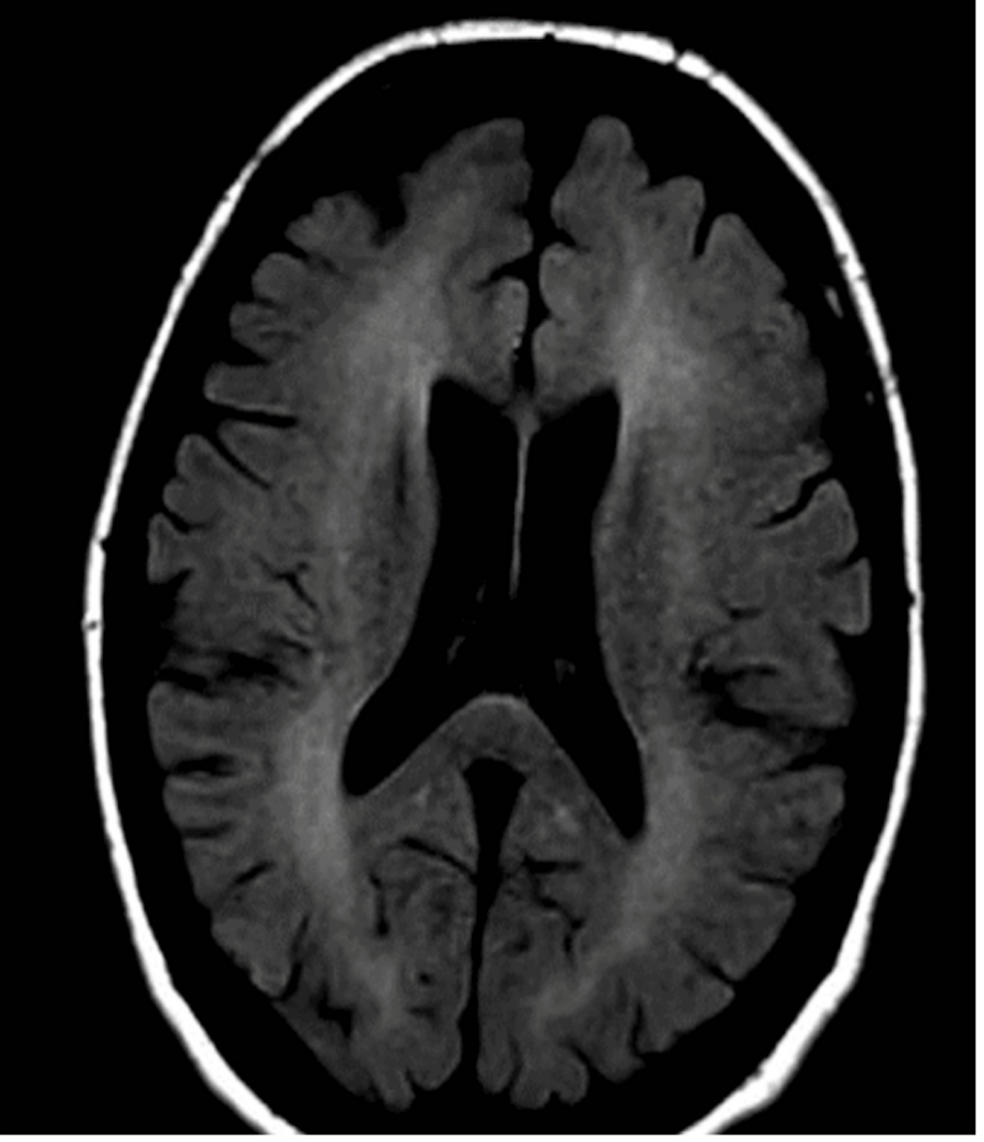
Brain MRI showing extensive periventricular leukoencephalopathy, characteristic for opioid inhalation.
